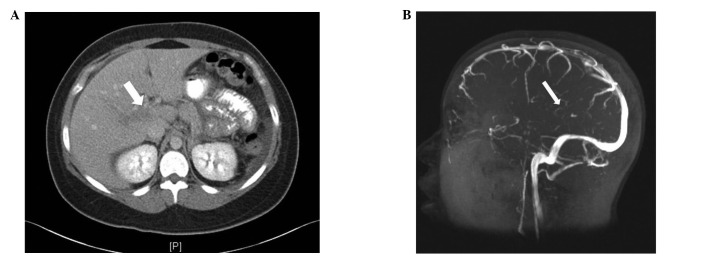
(A) Contrast abdominal CT scan showing a hypodensity and the absence of intravenous contrast noted in the area of the portal vein (white arrow). (B) MRV showing the absence of significant flow in the straight sinus, indicating a thrombosis (white arrow). CT, computed tomography; MRV, magnetic resonance venography.
